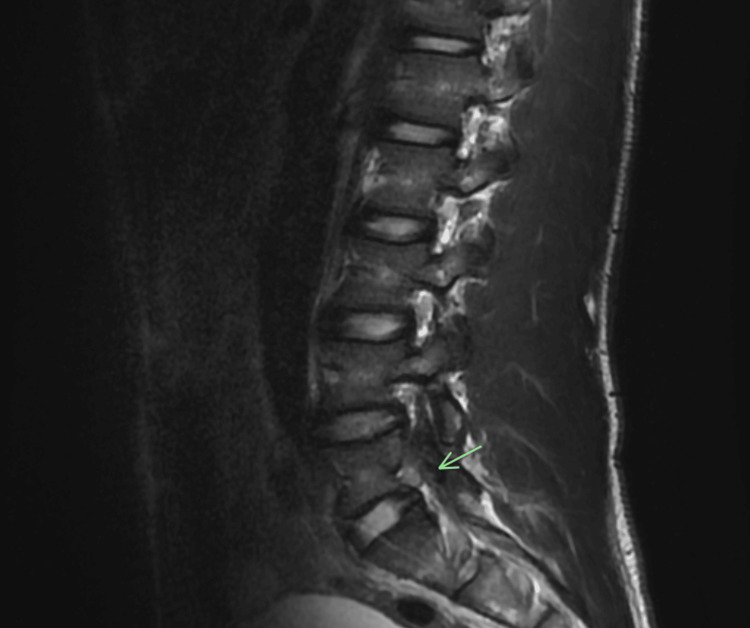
Sagittal T2-weighted MRI of the lumbar spine demonstrating moderate left-sided neural foraminal narrowing at the L5–S1 level. The green arrow indicates foraminal narrowing at L5/S1.
